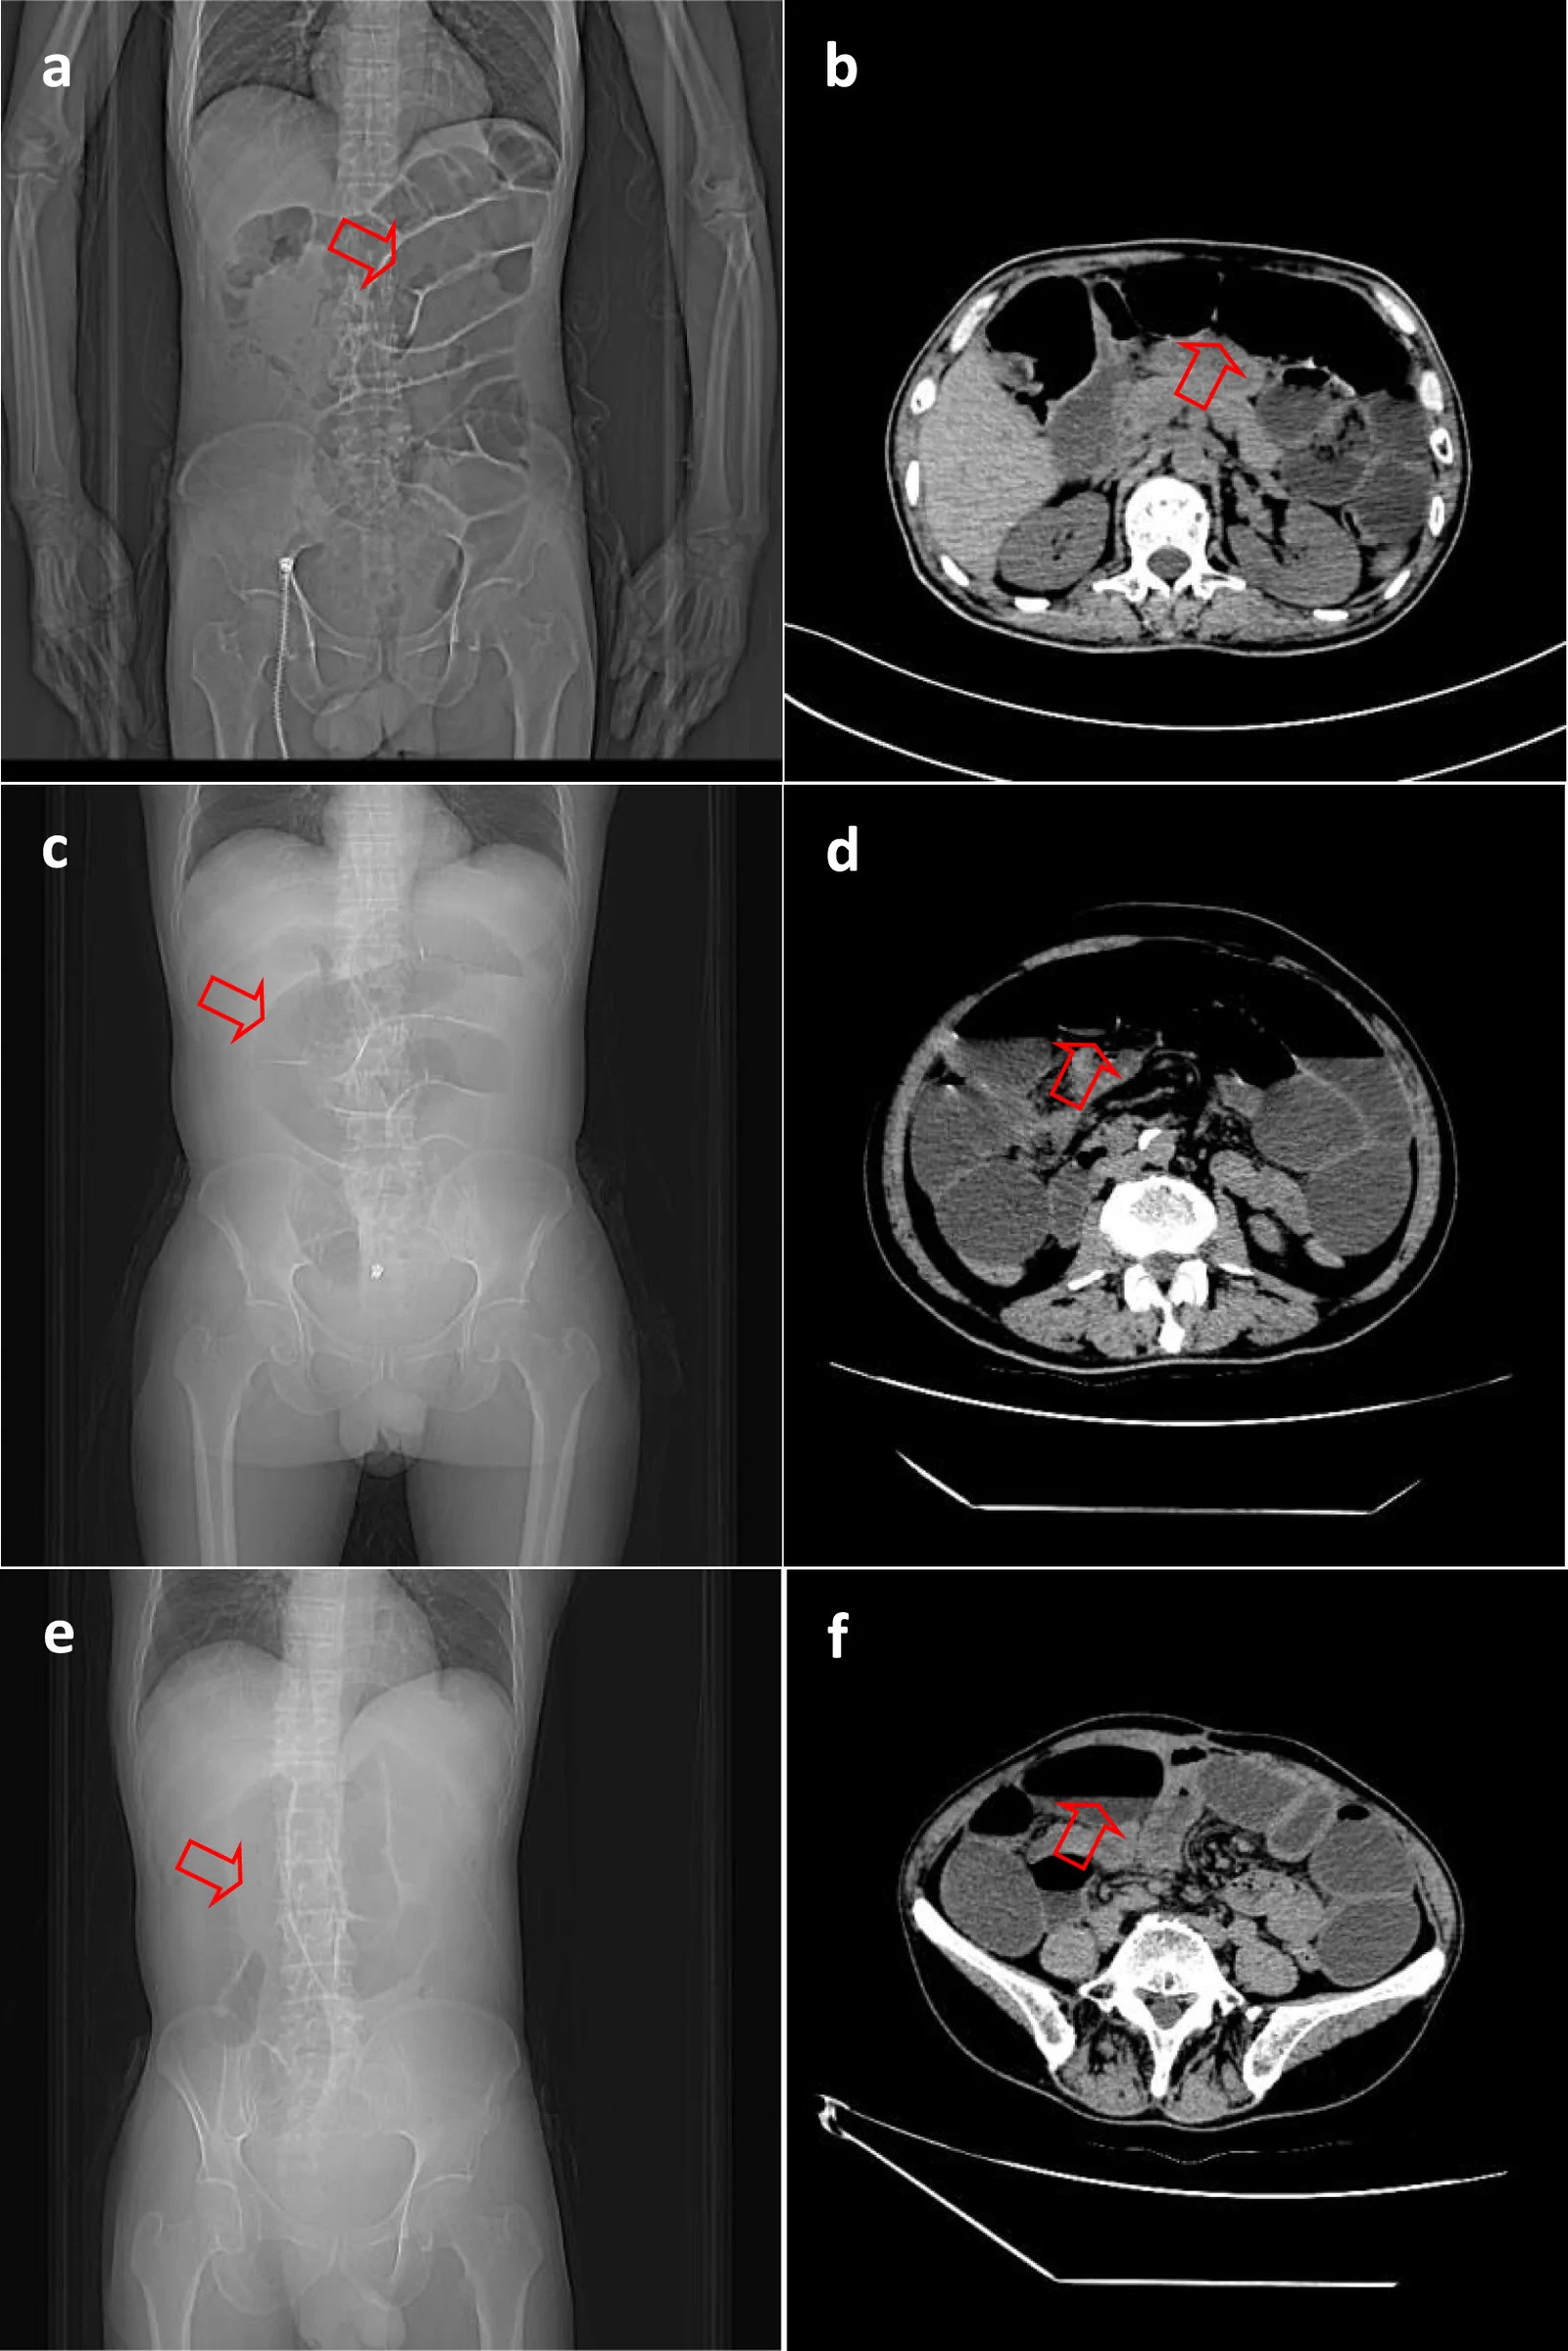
(a,b) CT, 30 November 2022. Abdominal intestinal dilation and gas accumulation, with multiple small fluid planes visible within the small intestine; (c,d) CT, 10 April 2024, Vortex sign is observed in the distal segment of the ileum and surrounding mesentery, with fluid accumulation and dilation in the upstream small intestine and visible gas-liquid plane; (e,f) CT, 6 February 2025. The mesenteric blood vessels show vortex like changes, and the small intestinal tract is dilated with fluid accumulation and a wide gas-liquid plane can be seen.
